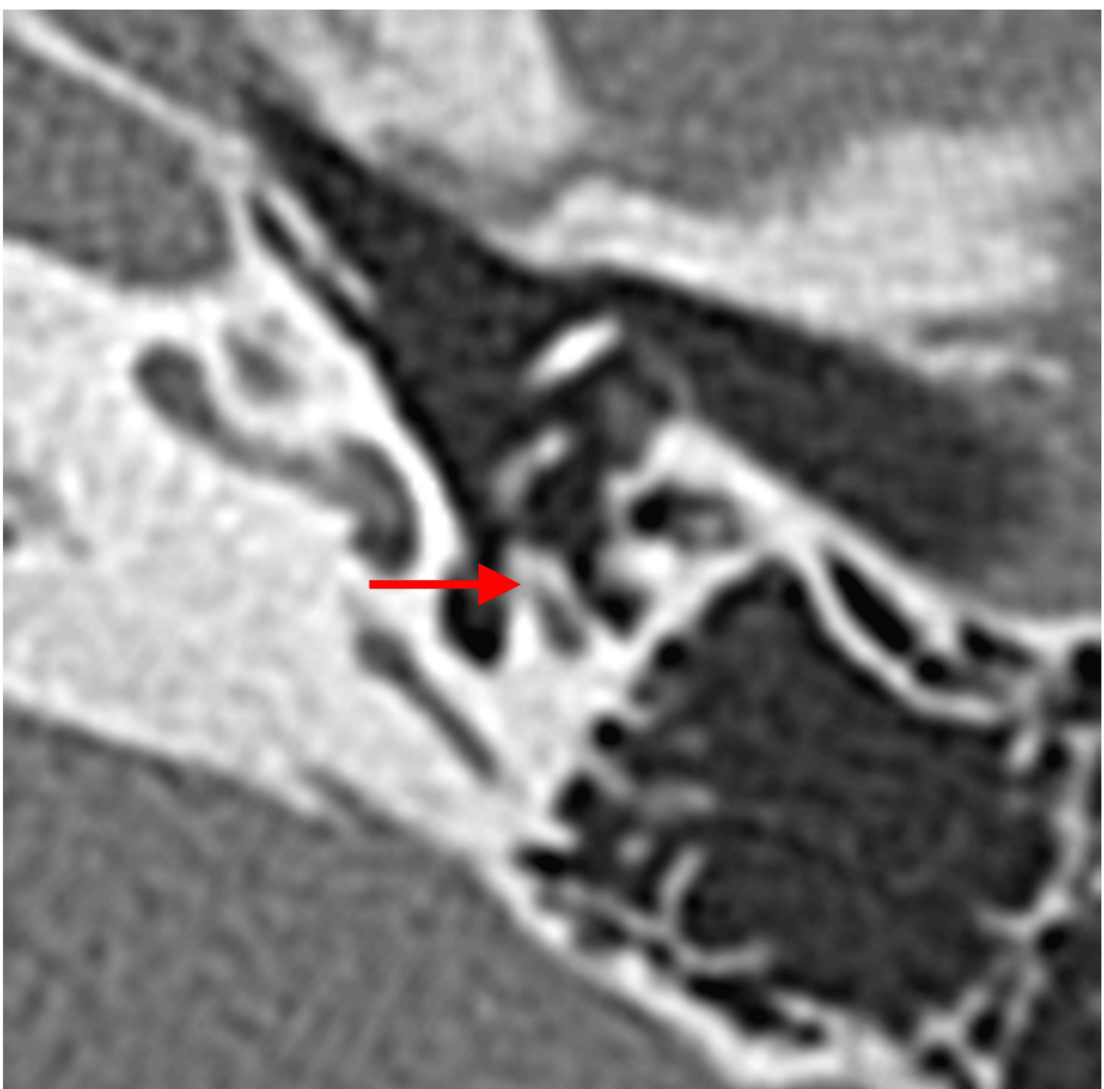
Axial HRCT image of the right temporal bone (Case 2) showing a normal pyramidal eminence (arrow). HRCT, High-resolution computed tomography
